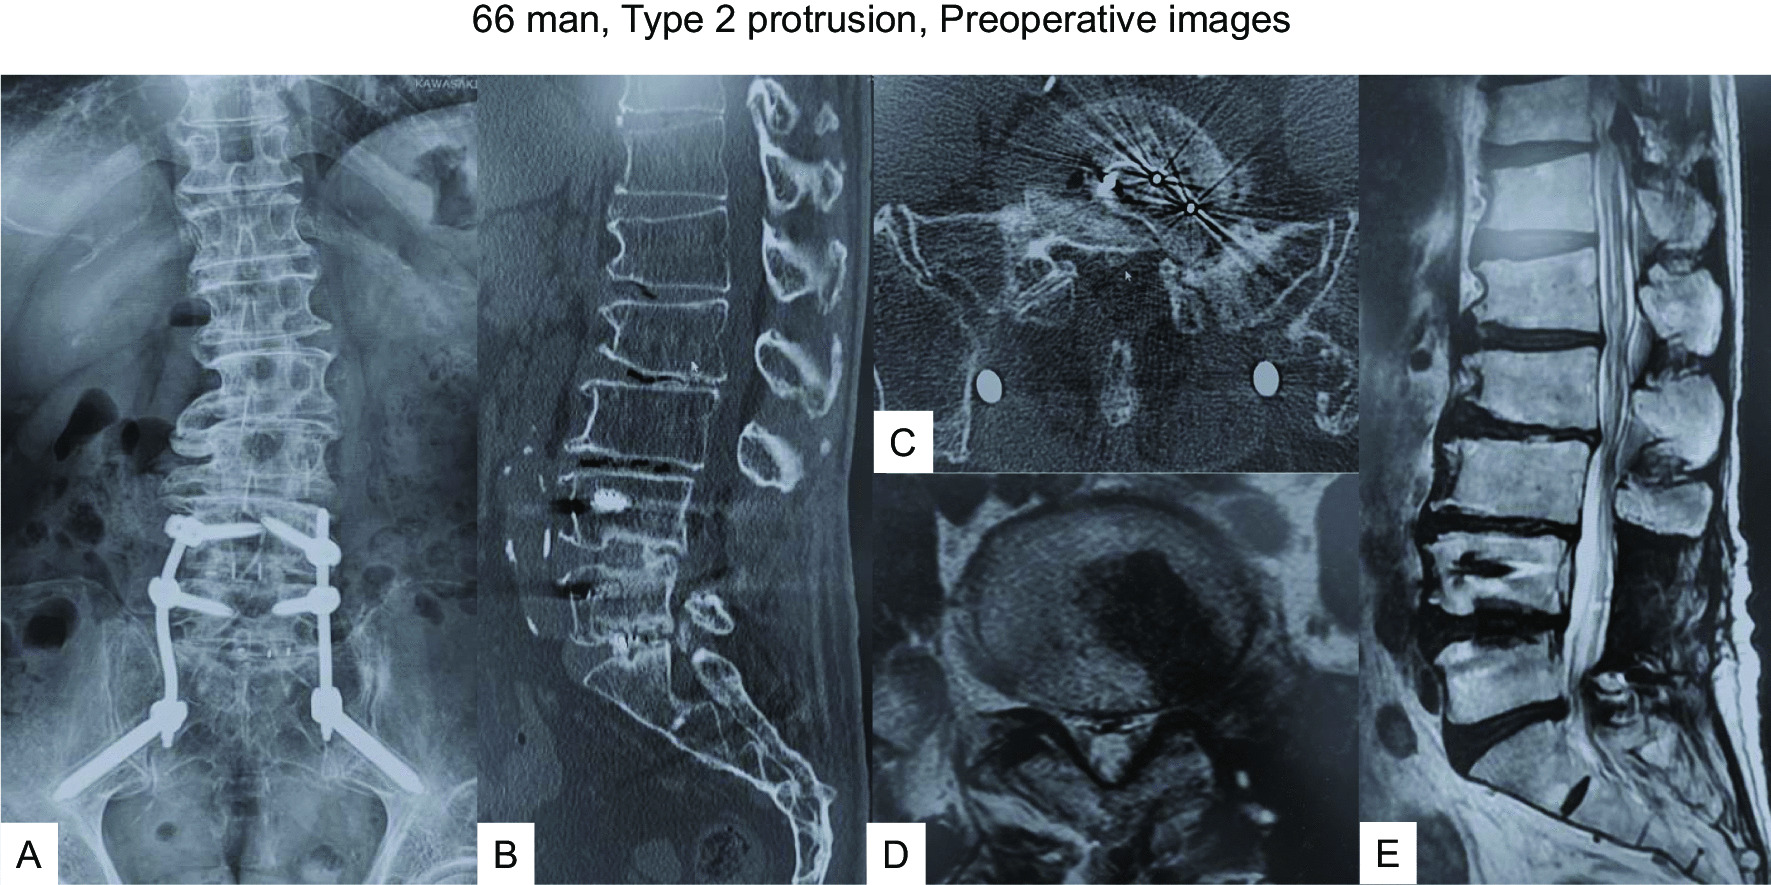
66 man, Type 2 protrusion, preoperative images A–E: Images at 5 months follow-up, A Anteroposterior radiogram, B Sagittal reconstruction CT, C Axial CT at L5/S1, D Axial T2-weighted MR imaging at L5/S1, E Sagittal T2-weighted MR imaging
